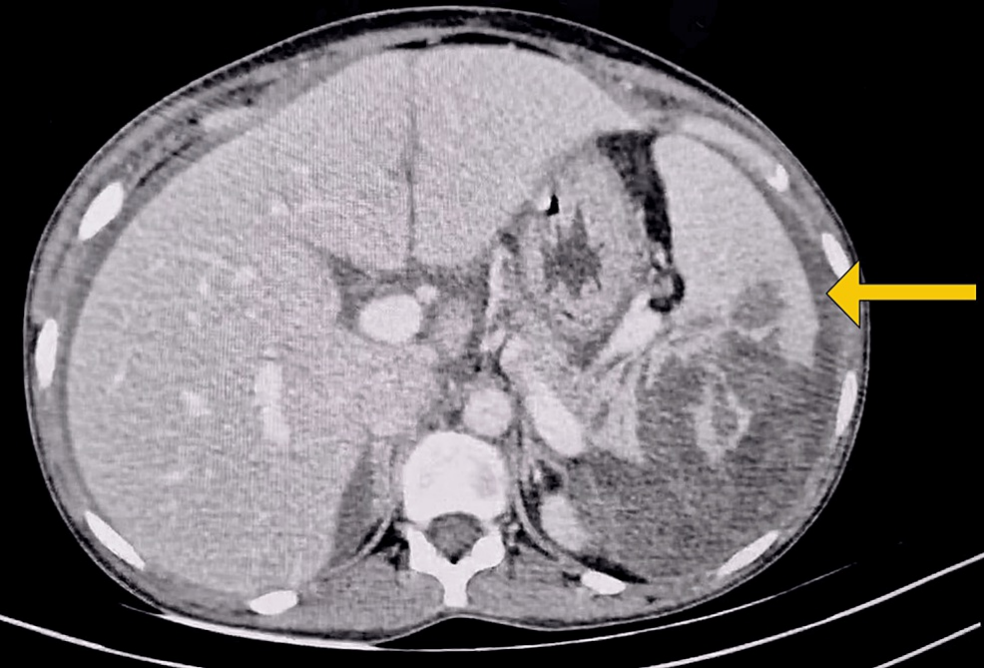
CECT of abdomen Splenic rupture with hemoperitoneum (indicated by a yellow arrow) CECT: Contrast-enhanced computed tomography
